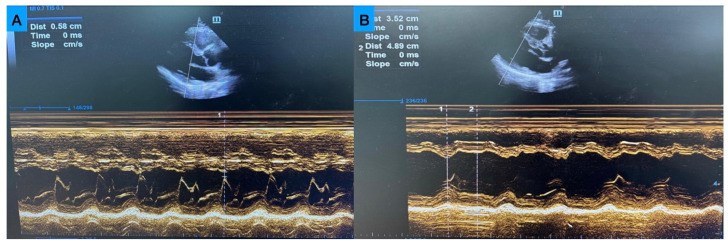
Measurement of E-point septal separation (EPSS) and fractional shortening (FS) using point-of-care ultrasound (POCUS). (A) EPSS was measured in early diastole on parasternal long-axis (PLAX) M-mode POCUS as the distance between the tip of the anterior mitral valve leaflet and the interventricular septum. (B) FS was calculated from M-mode images obtained at the mid-ventricular level in PLAX, with the ultrasound beam positioned perpendicular to the interventricular septum and posterior wall. Left ventricular internal diameters at end-diastole (LVIDd) and end-systole (LVIDs) were measured, and FS was derived as FS = (LVIDd − LVIDs)/LVIDd × 100.
